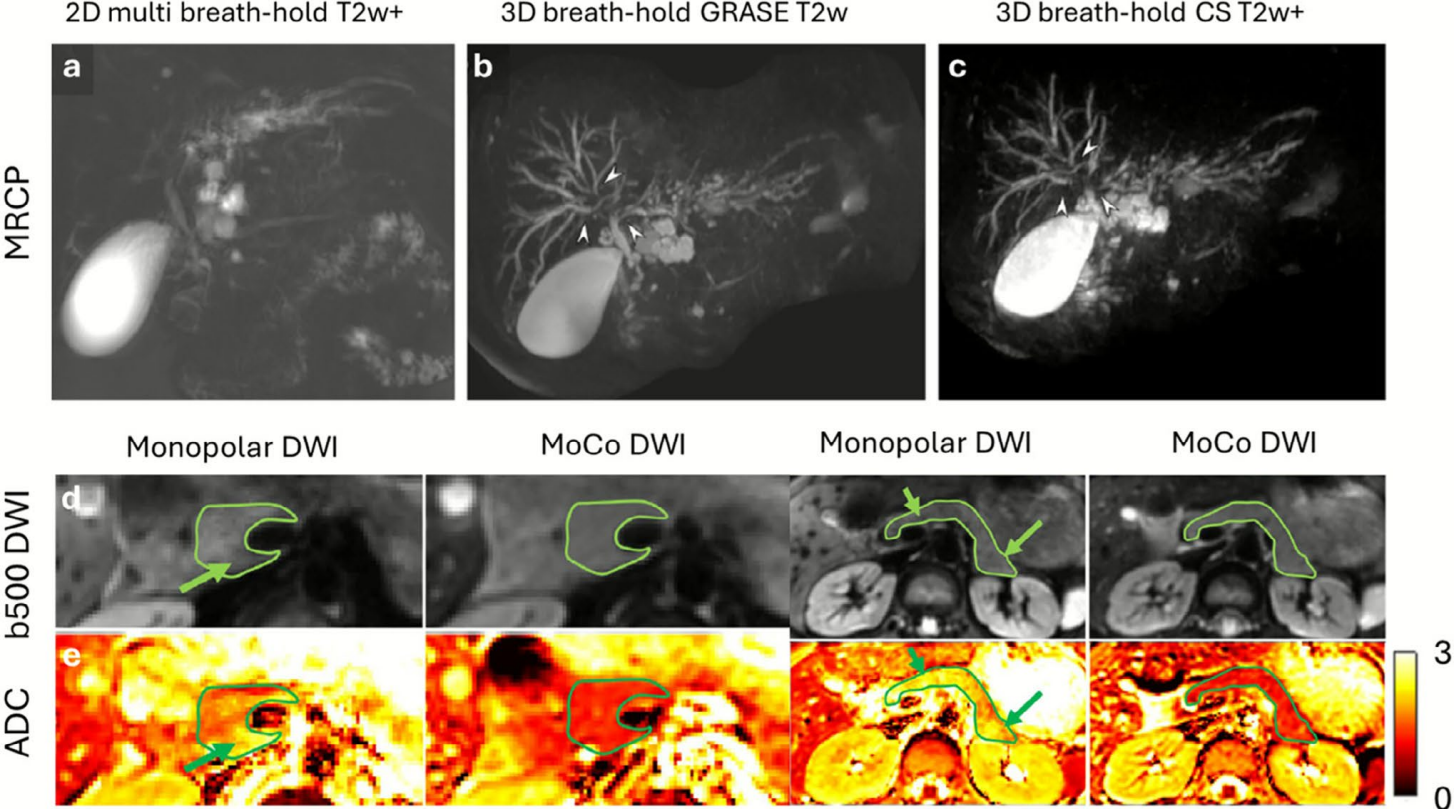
(a–c) Multi breath‐hold T2w + (A) failed likely due to inconsistencies between breath‐holds; CS allowed to accelerate MRCP scans by 10–20×, enabling 3D T2w + single breath‐held MRCP. (d and e) Effects of cardiac pulsation on pancreatic diffusion‐weighted imaging (DWI), in which signal dropout is observed with monopolar diffusion gradients. Homogeneous DWI and ADC signal is observed with the application of velocity compensated diffusion gradients. Adapted from [89, 90].
